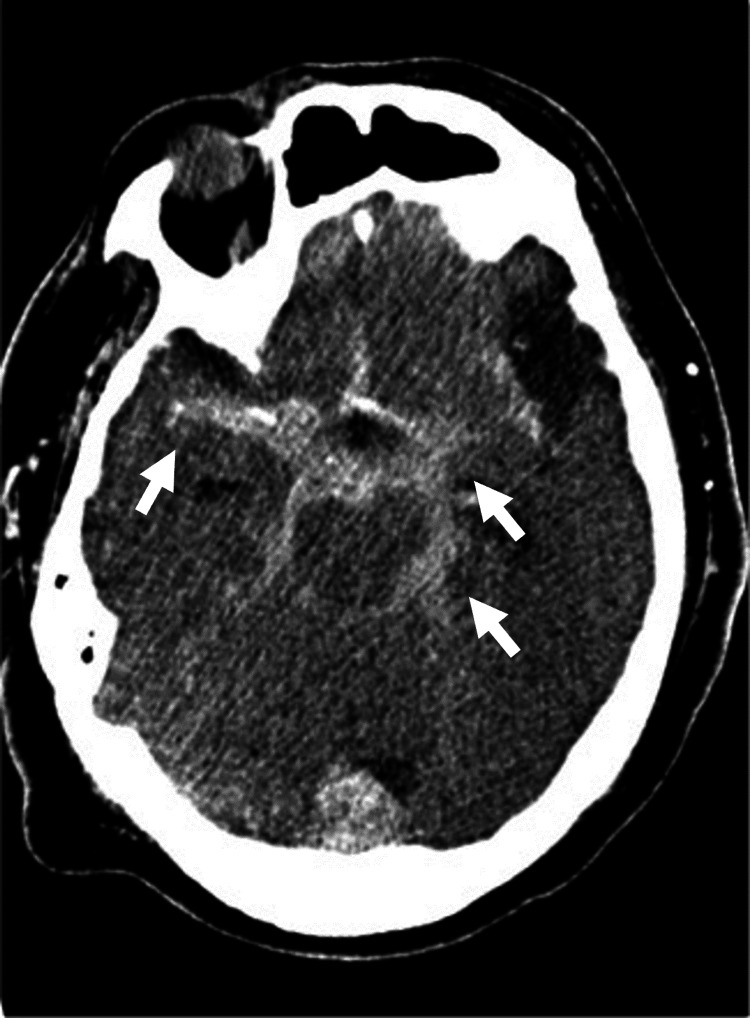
Computed tomography (CT) scan of the head performed when the patient's condition suddenly changed. The white arrows indicate hemorrhage extending into the basal cistern, ambient cistern, and Sylvian fissure, consistent with a diagnosis of acute subarachnoid hemorrhage (SAH).
